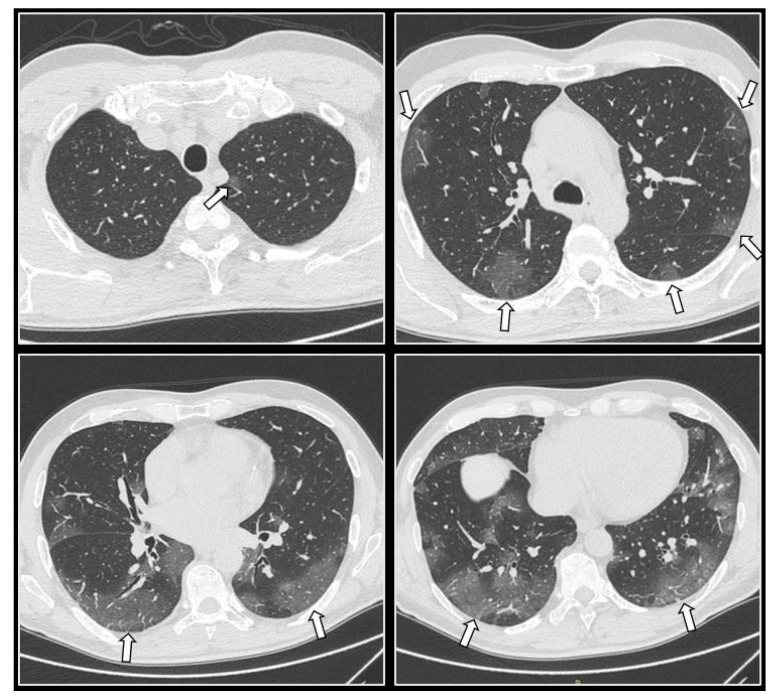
Axial images from a chest CT of a COVID-19 patient demonstrate multiple ground glass opacities (arrows) in both lungs and multiple lobes which are primarily distributed peripherally, posteriorly, and in the lower zones. Opacities occupy approximately 26–50% of the total lung parenchyma according to visual assessment [45].
